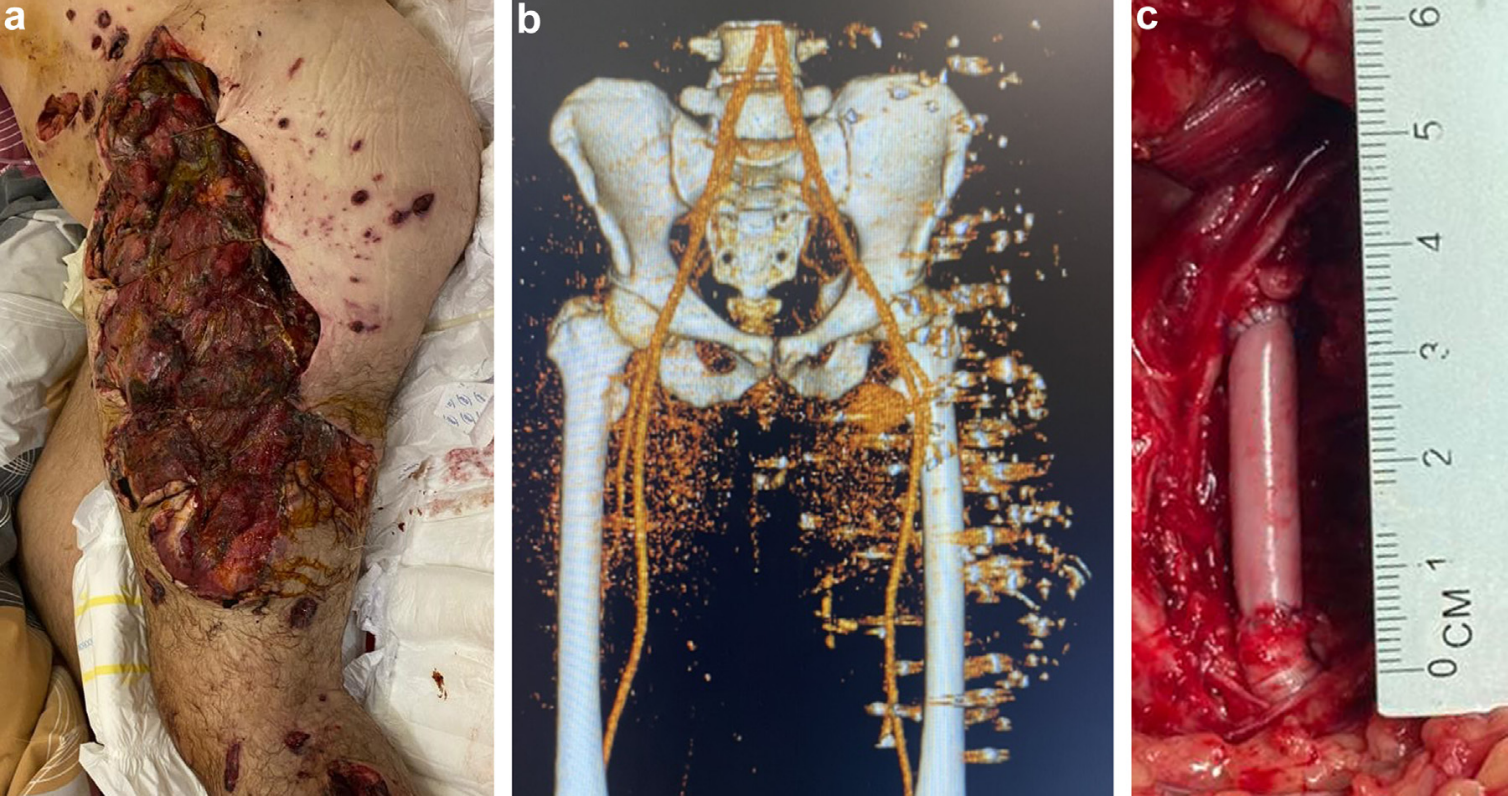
War-wounded patient in Ukraine. a. 29-year-old male suffered blast injury to the left leg; b. Pre-operative CT scan shows shrapnel fragments, including in vascular bundle near inguinal ligament; c. Repair of left common femoral artery with HAV, which has resisted infection during follow-up.
